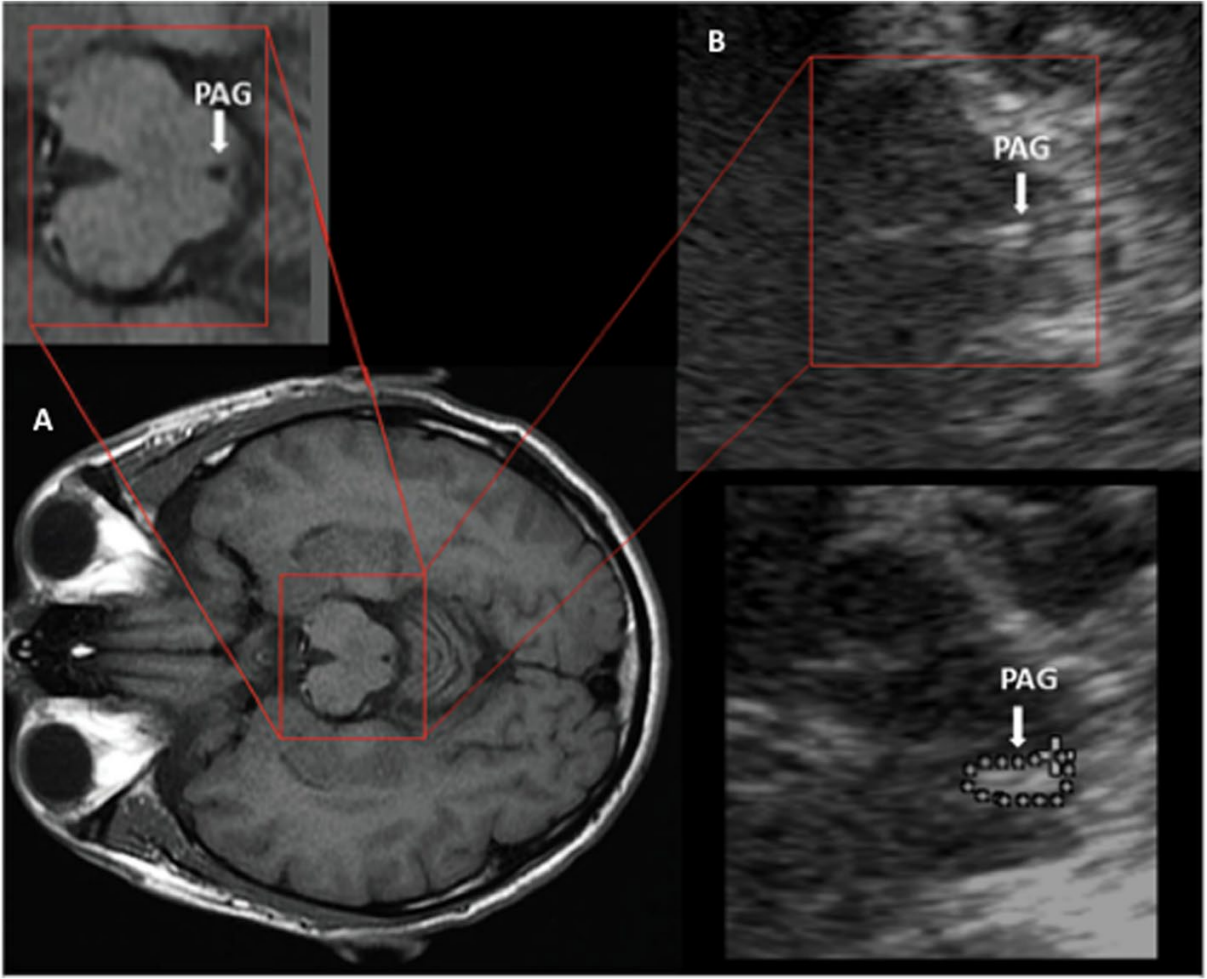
Magnetic resonance and transcranial sonography images of midbrain axial sections showing the periacueductal gray matter. The TCS images show images from our sonographic laboratory obtained with an ultrasound system for clinical application (Philips Affiniti 70 ultrasound machine). A MRI of the axial section at the level of the midbrain. The square box shows the area corresponding to the magnified TCS image shown in B. B TCS image of the axial midbrain section showing the area of the periaqueductal gray matter echogenicity
